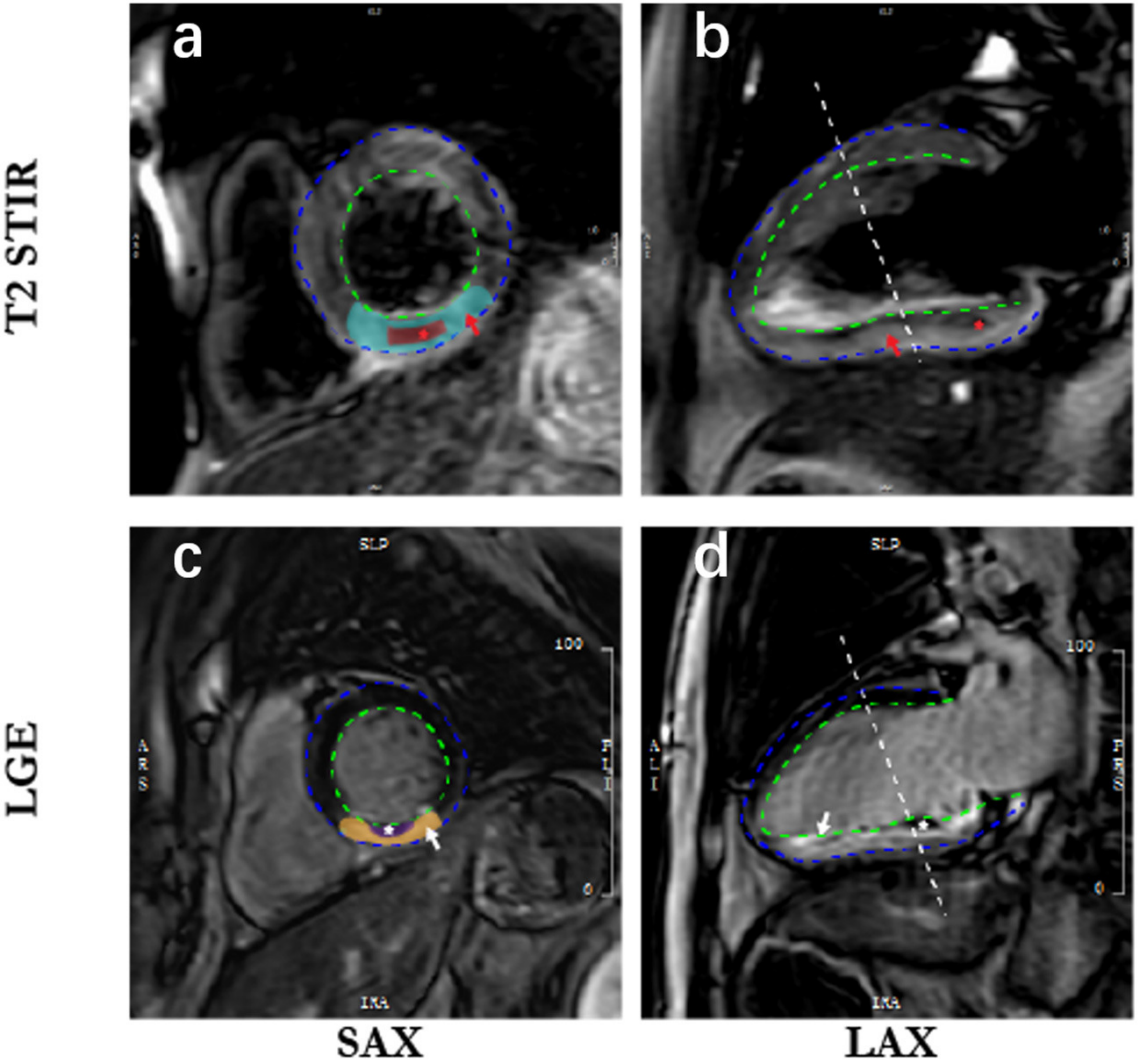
Cardiac magnetic resonance imaging in patients with acute myocardial infarction. SAX, short axis; LAX, long axis; LGE, late gadolinium enhancement. The blue dotted line is epicardium; the dotted green line is endocardium; (a) indicates myocardial edema (blue-green zone) defined as high-signal myocardium within the territory of the culprit's vessel; (b) shows the intramyocardial hemorrhage (red star) in the hyposignal region within the edema; (c) is infarction (yellow zone) defined as hyperenhanced myocardium, and corresponding infarct size is quantified and normalized to the left ventricle mass; 1 (d) represents microvascular obstruction (white zone) defined as a hypo-enhanced area within the infarcted-related zone.
